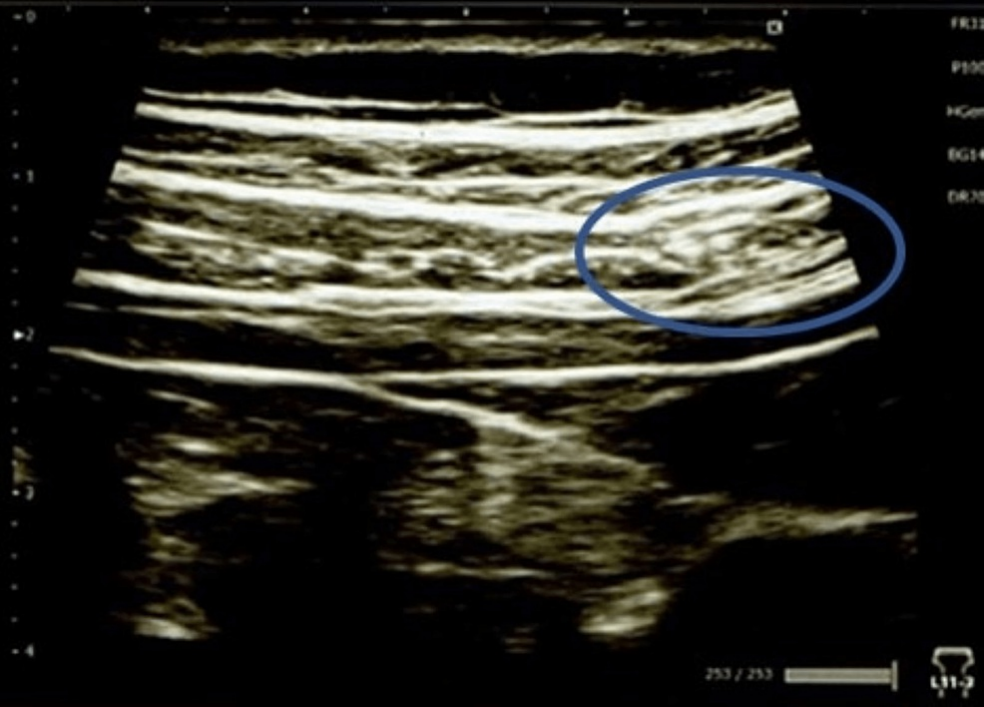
Ultrasonographic findings on the first visit A 5 × 10 mm mass shadow was observed in the internal oblique muscle, which coincided with the strongest pain point.
